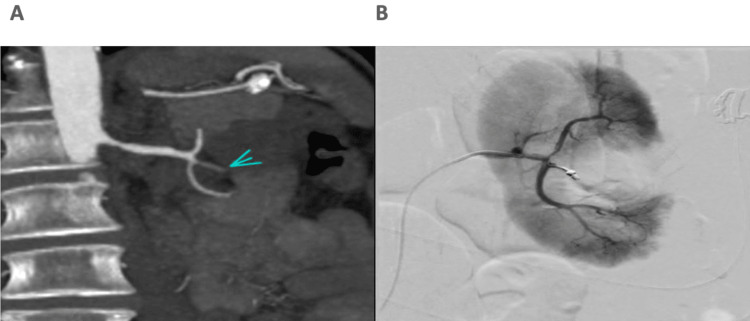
Left renal hematoma with pseudoaneurysm and post-embolization image (A) CT angiography demonstrating the pseudoaneurysm and the hematoma in the left kidney. (B) Digital subtraction angiography of the same area post-embolization CT: computed tomography
